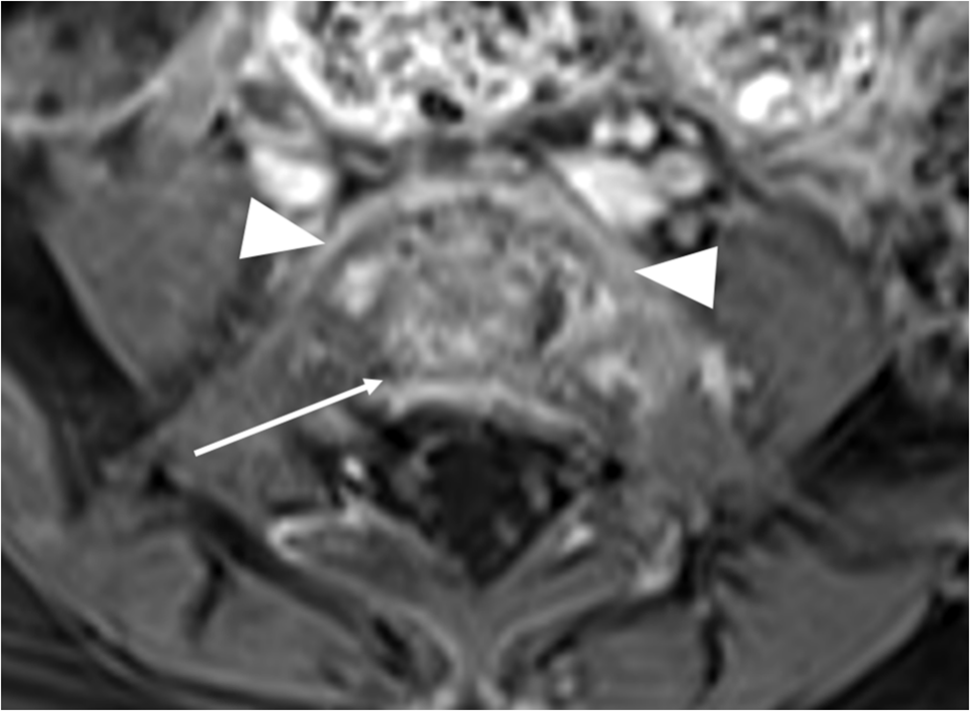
Axial contrast-enhanced T1-weighted magnetic resonance image of the lumbar spine in a 21-month-old girl with spondylodiscitis, 1 month after initial diagnosis, demonstrates complete resolution of the epidural collection (*arrow*) and near-complete resolution of the paravertebral soft tissue enhancement (*arrowheads*)
